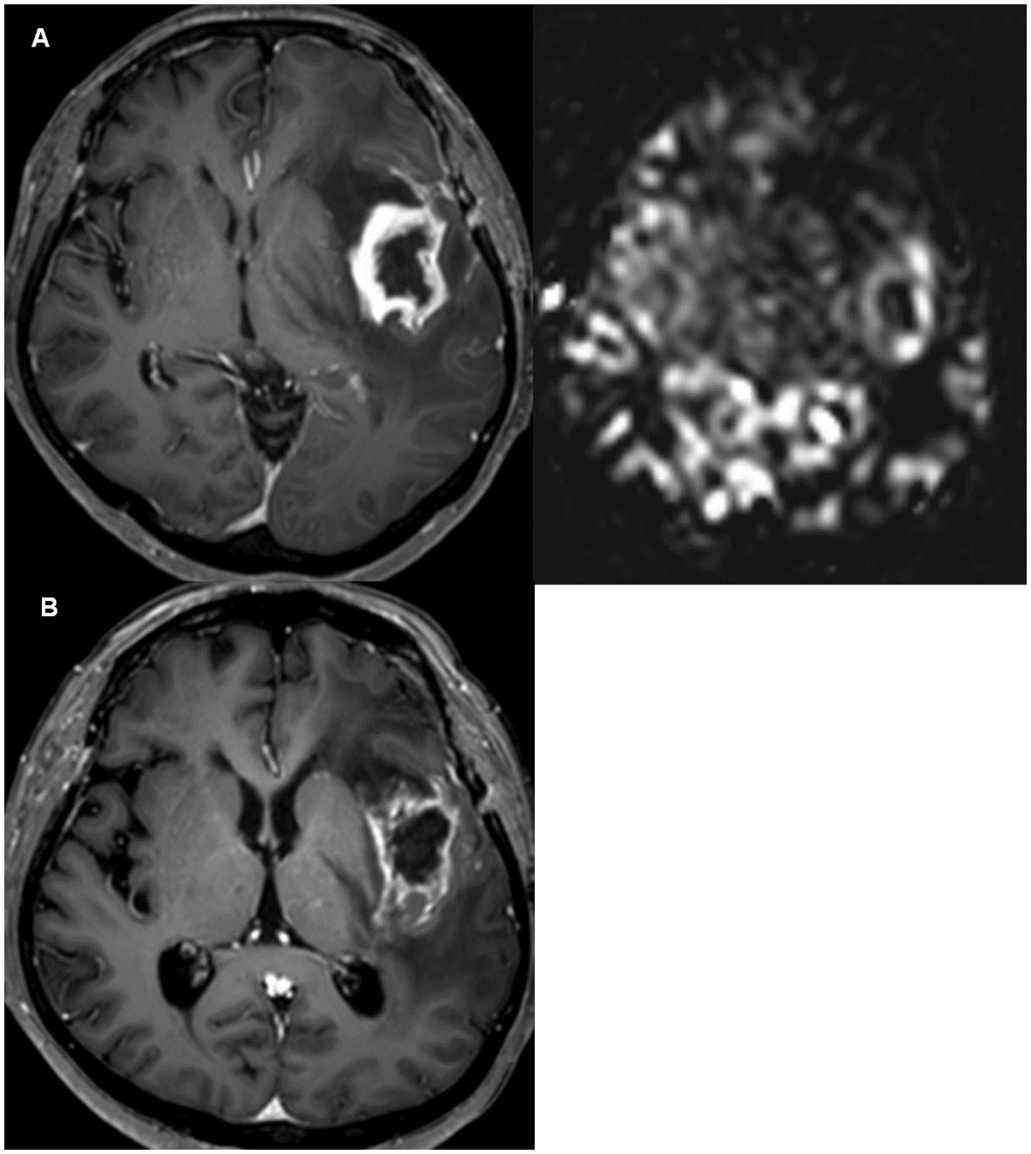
A*, MR images from a 53-year-old male patient with glioblastoma taken 3 weeks after concurrent chemo-radiation. The contrast-enhanced MRI (left) and ASL (right) showed positive perfusion along surgical cavity wall enhancement. *B*, Follow-up images taken 4 months later after additional 5 cycles of temozolomide show that the SCWE becomes stabilized.
